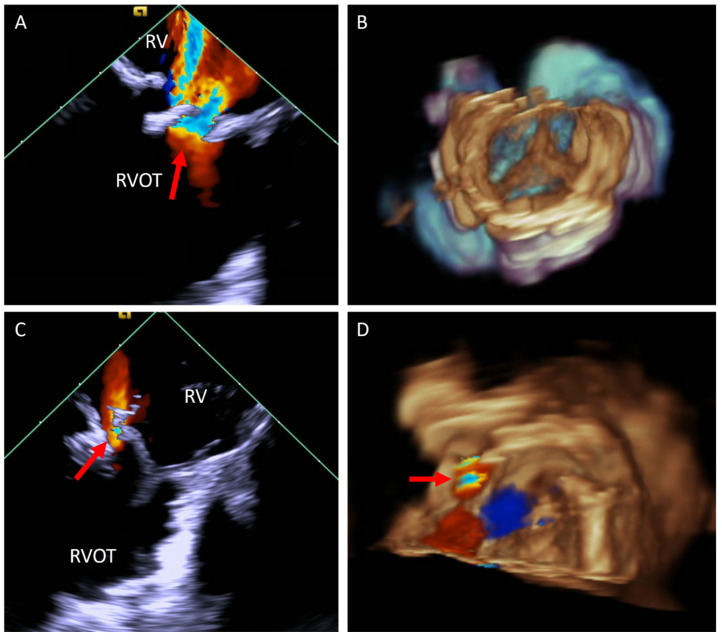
ICE catheter in the right ventricle. (A) Color Doppler image at the RVOT showing severe pulmonary regurgitation (arrow). (B) Three-dimensional image of a valve post TCPVR showing the three valve leaflets. (C,D) Color Doppler of the RVOT in 2D (C) and 3D (D) post TCPVR showing mild paravalve leak (arrow) with the 3D image showing the exact location of the paravalve leak. ICE, intracardiac echo; RVOT, right ventricular outflow tract; TCPVR, transcatheter pulmonary valve replacement.
